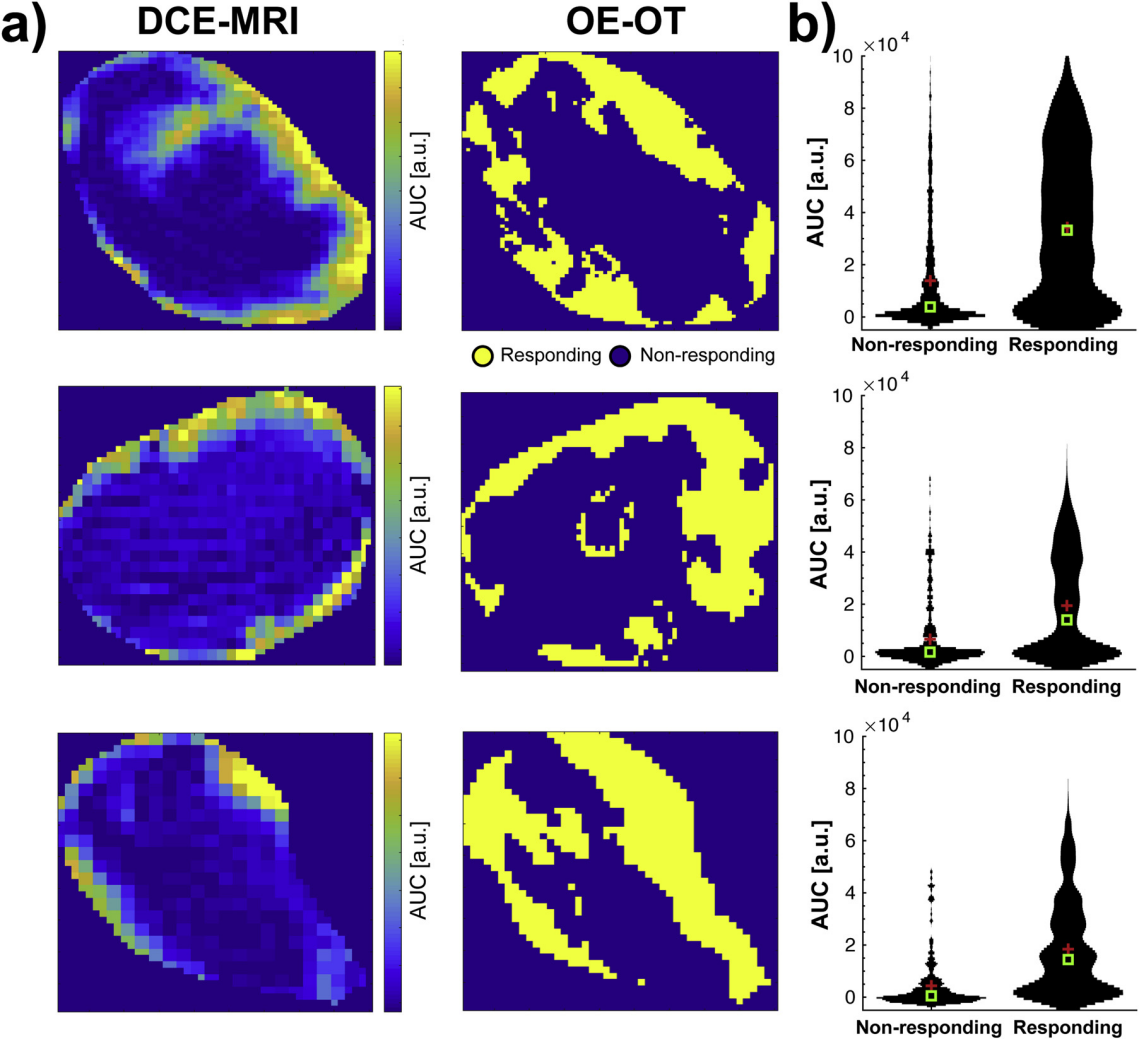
Measurements of vascular function show strong co-localisation in MRI and MSOT. (a) Maps of DCE-MRI area under the curve (AUC) enhancement 1 min after contrast injection (left) are in close spatial agreement with the maps of positive response to oxygen challenge in Oxygen-Enhanced Optoacoustic Tomography (right). (b) The DCE-MRI AUC is clearly higher in the areas positively responding in OE-OT. Mean and median are shown as red crosses and green squares, respectively. The width of the black lines indicates the distribution of values.
